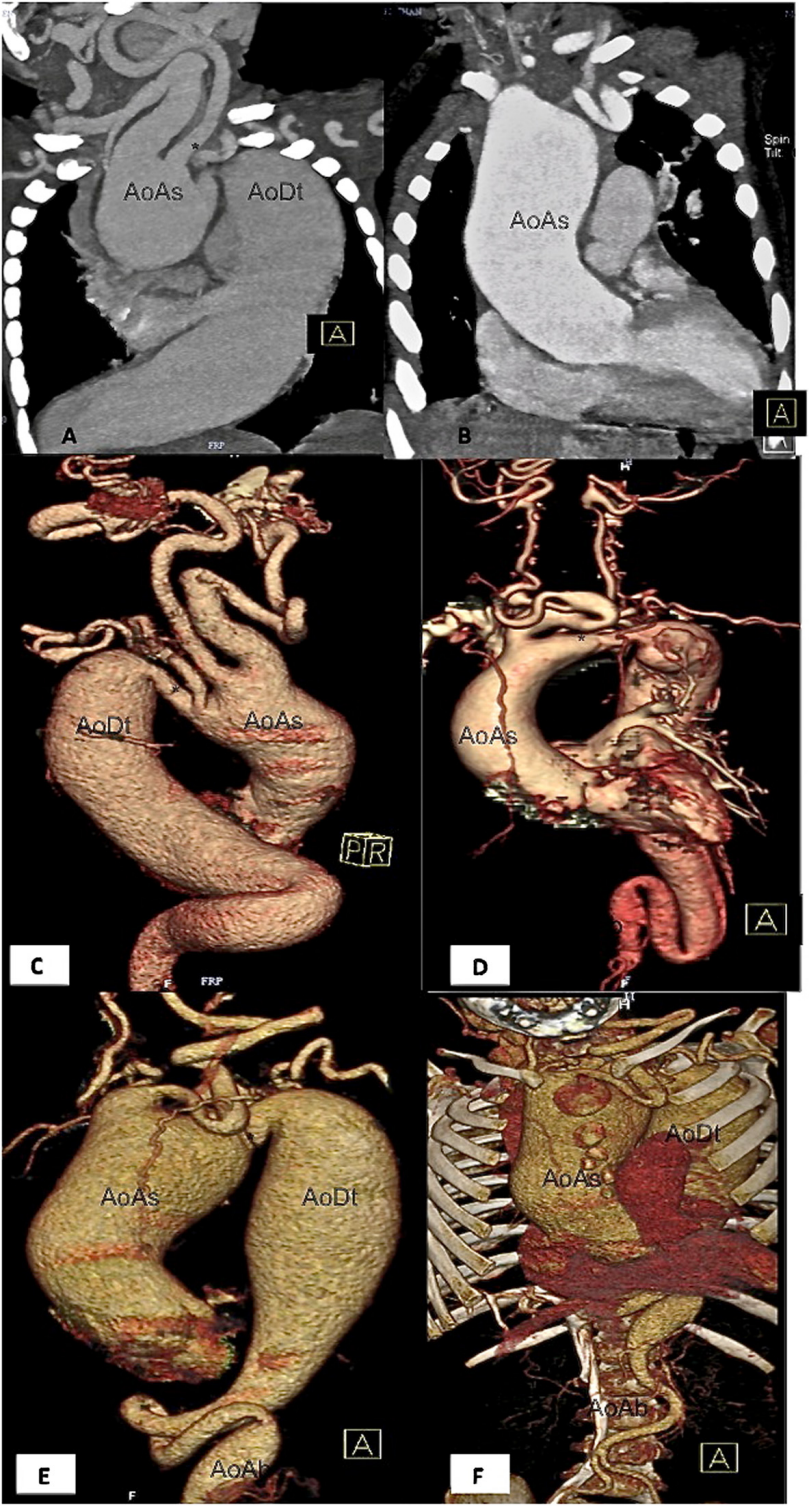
Cardiovascular imaging.A- F : 64-slice cardiac CT
